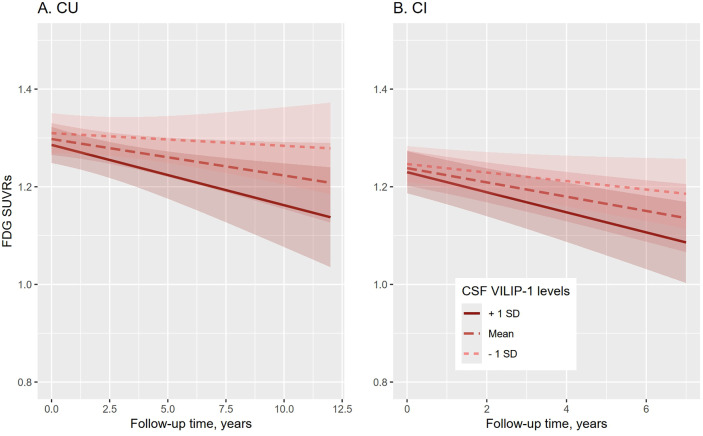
Association of CSF VILIP-1 levels with changes in cerebral glucose metabolism across cognitive status. Abbreviations: CU: cognitively unimpaired; CI: cognitively impaired; FDG: fluorodeoxyglucose; SUVRs: standardized uptake value ratios; VILIP-1: visinin-like protein 1.
